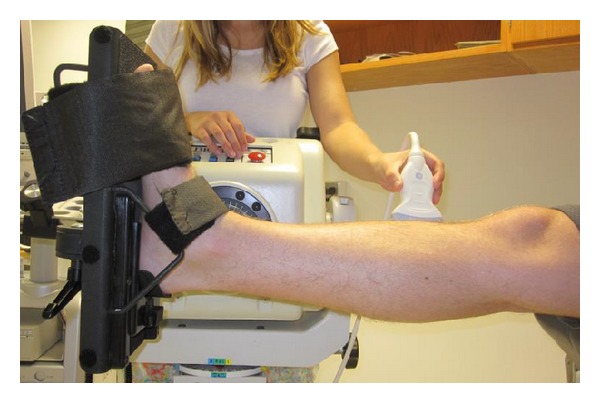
Subject in isometric dynamometer with ankle secured in neutral position. Ultrasound images were taken at the midbelly of the tibialis anterior. Care was taken to keep the probe perpendicular to the skin. Enough pressure was applied to maintain proper contact between the probe and skin without significantly deforming the muscle.
